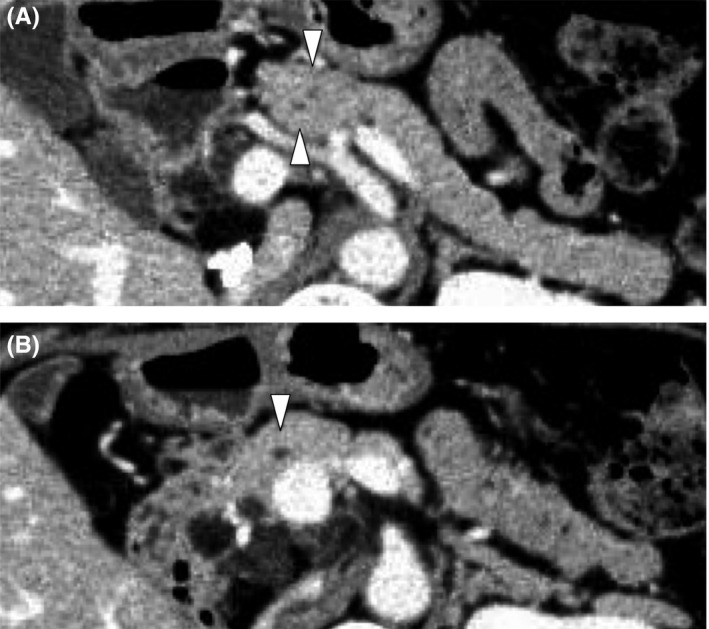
Preoperative multi‐detector computed tomography shows a bifurcated double main pancreatic duct. A, There were two pancreatic ducts in the pancreas body. B, Two ducts joined at the level of the portal vein. Arrowheads in part A shows double main pancreatic duct. Arrowhead in part B shows the main pancreatic duct on the left side of the confluence of double pancreatic duct
